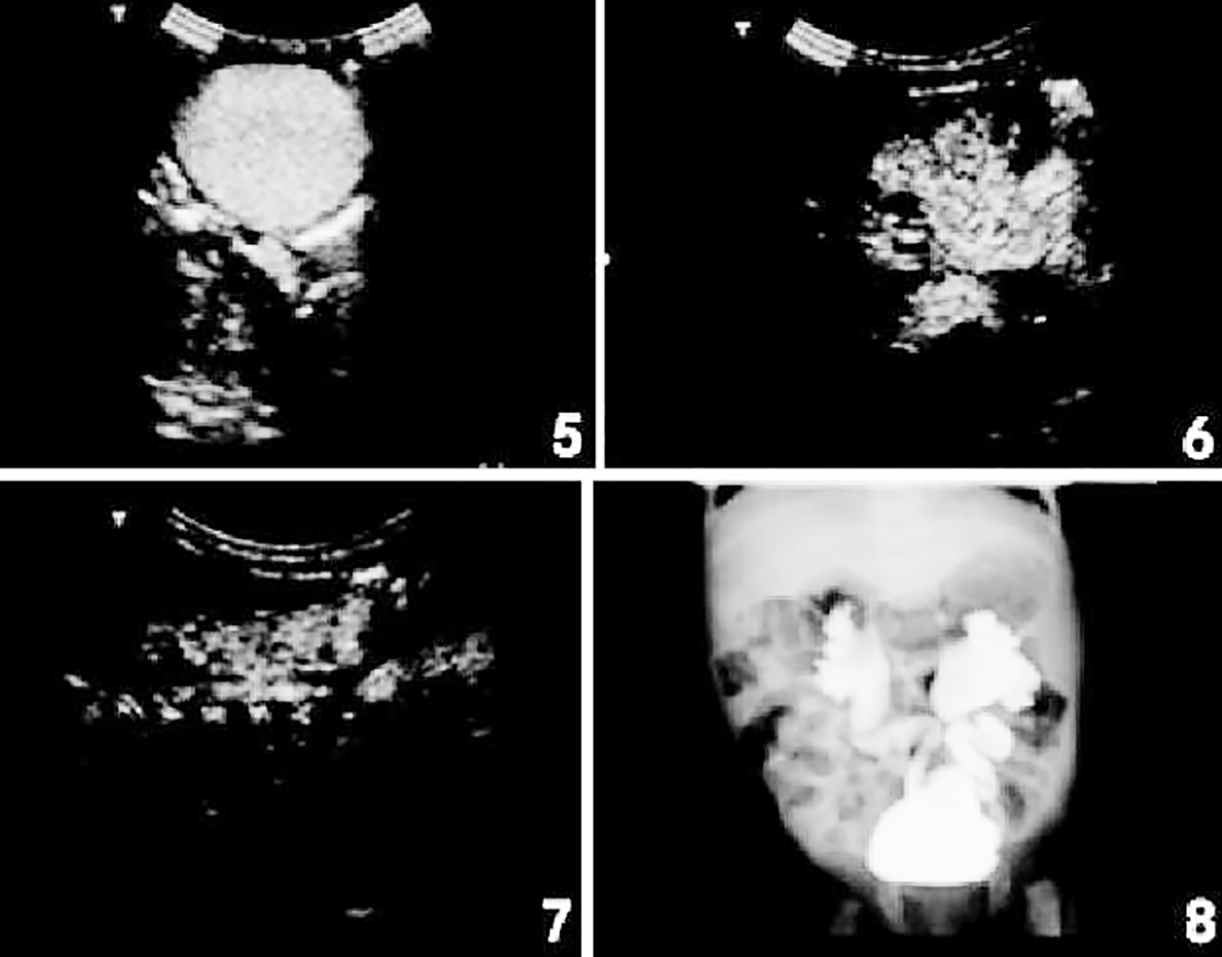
The diagnostic results of contrast-enhanced cystosonography and VCUG.
